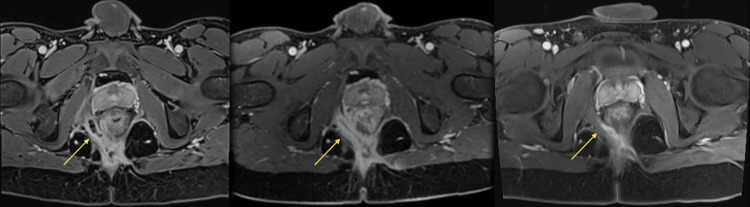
Serial axial T1-weighted contrast-enhanced MRI of the perianal region showing interval improvement of a curvilinear fluid-filled track (arrow) in the right ischioanal fossa over time. This track was one component of a complex perianal fistula with multiple openings. A (left): Appearance at first presentation. B (middle): Two months post treatment. C (right): Five months post treatment.
